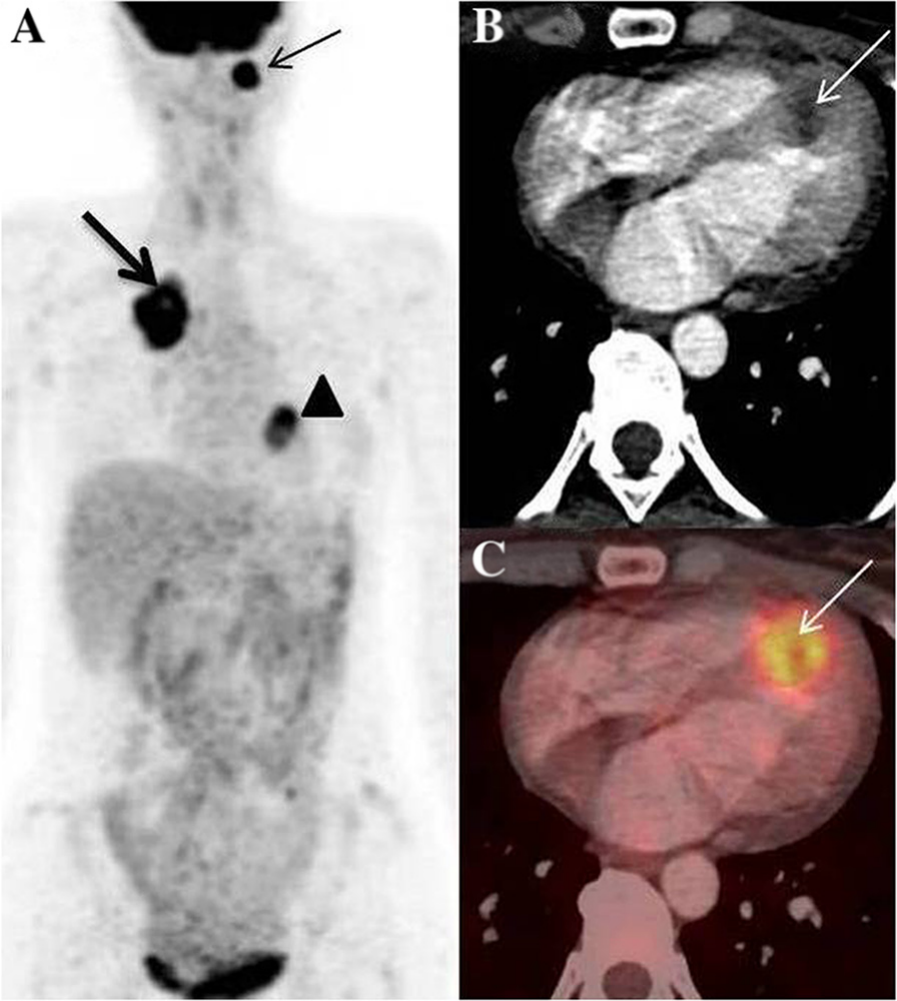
Myocardial metastases from tongue cancer. A - MIP image showing tracer uptake in left neck region (thin arrow), with another large area in thorax (thick arrow). Focal uptake is noted in cardiac region (arrow-head). B - large hypodense lesion in left ventricular myocardium, C - FDG uptake in the myocardial mass.
